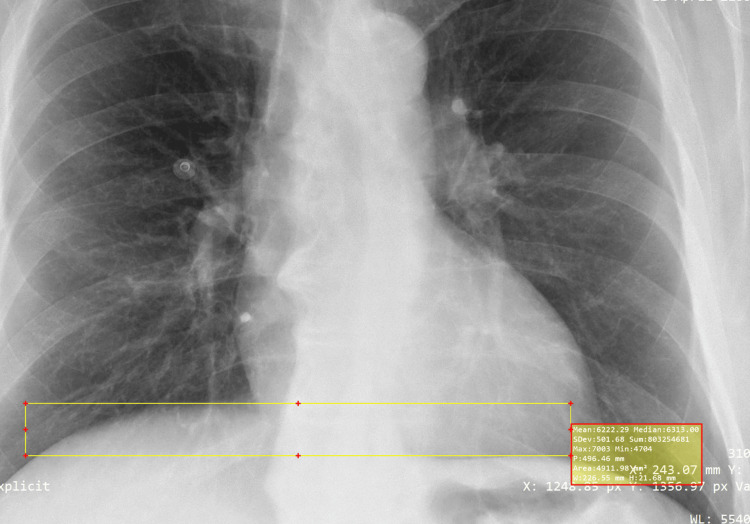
Frontal CXR projection obtained from the MIMIC-IV CXR database utilizing the DICOM Bee software and the box technique while assessing for evidence of DE. Yellow parallel lines are placed at apex of both hemidiaphragms. Hemidiaphragm height differences measured at 2.4 cm. CXR from the MIMIC-CXR Database v2.0.0, a publicly available dataset developed by Johnson et al. at the Massachusetts Institute of Technology. Image used under a Creative Commons Attribution 4.0 International License (CC BY 4.0). Source: Johnson et al. [14] CXR, chest X-ray; DE, diaphragmatic eventration; MIMIC-IV, MIT Medical Information Mart for Intensive Care, Fourth Edition
